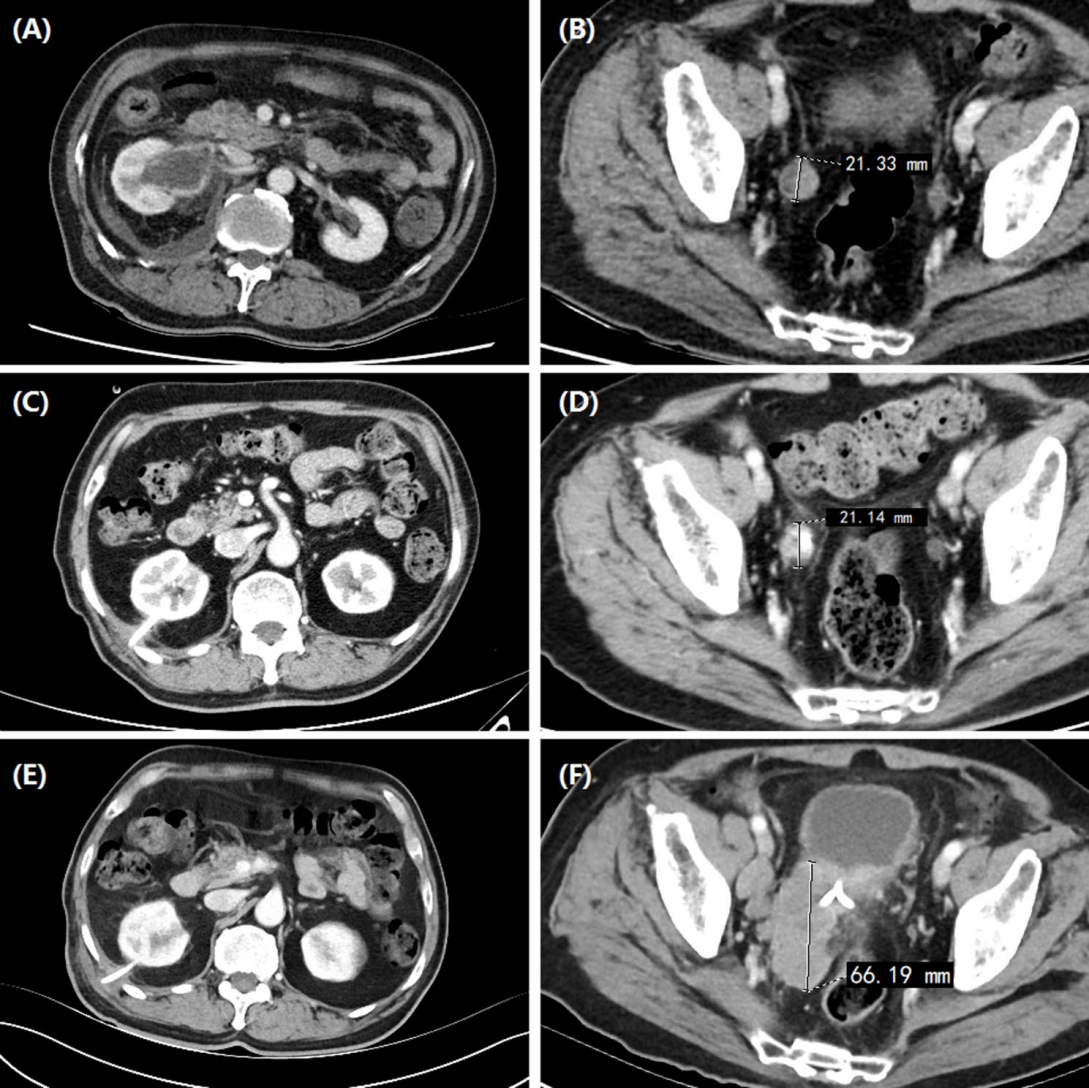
A 81-year-old male patient with right ureteral carcinoma. (A) The pretreatment CT showed obvious dilatation of the renal pelvis and hydronephrosis; (B) CT showed ureteral carcinoma (21.33 mm in diameter) in the lower segment of the ureter; (C-D) The posttreatment CT showed that the hydronephrosis disappeared after nephrostomy, and the tumor diameter was 21.14 mm about 3 months later; (E-F) The posttreatment CT showed PD after 4 years according to the tumor evaluation system;
